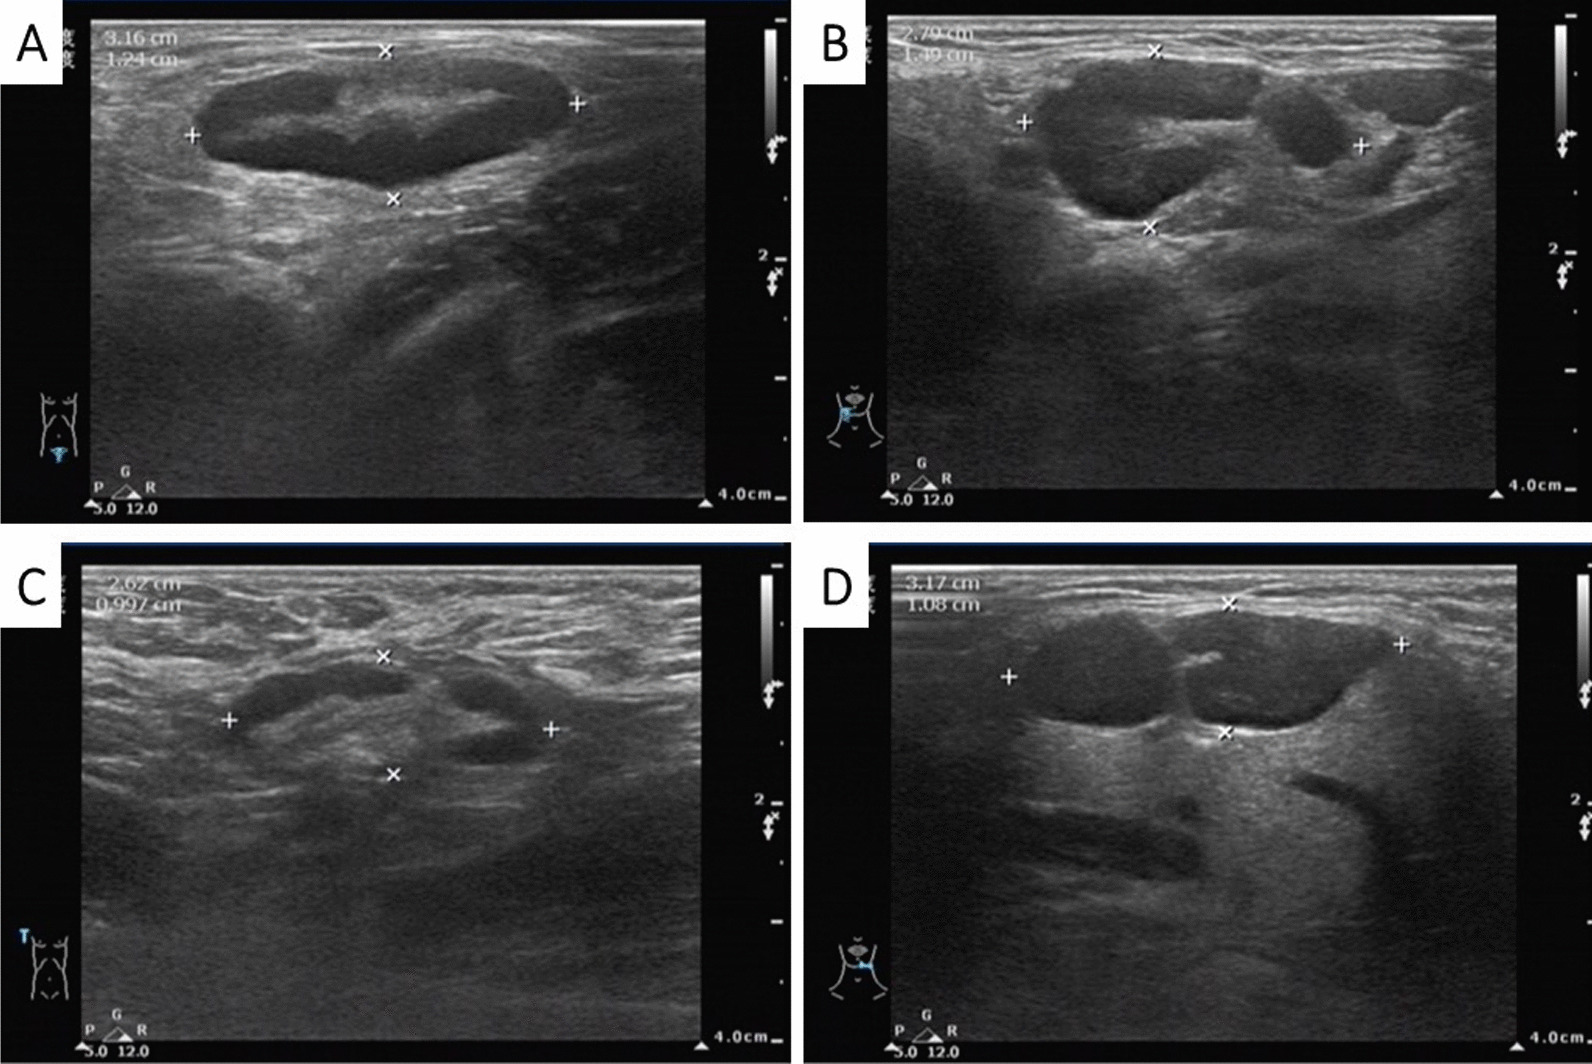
The enlargement of the superficial lymph nodes under B-ultrasonic examination. (A: inguinal lymph node 3.16 cm × 1.24 cm; B: submandibular lymph node 2.79 cm × 1.49 cm, C: supraclavicular lymph node 2.62 cm × 0.997 cm, D: Neck lymph node 3.17 cm × 1.08 cm)
